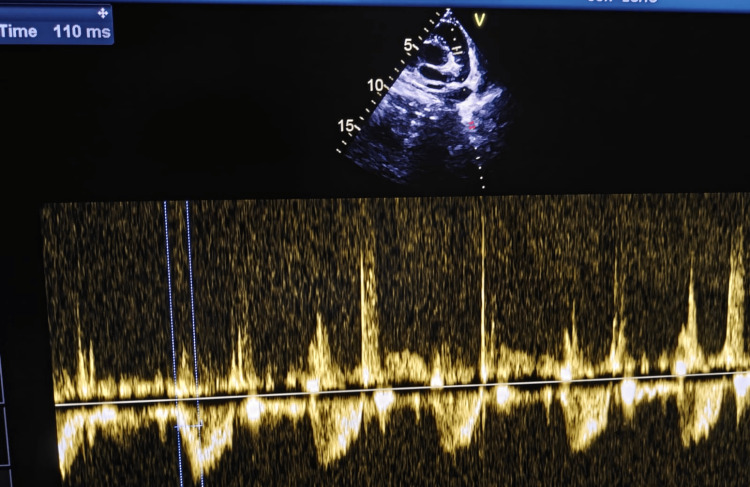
2D echo: high parasternal short axis (PSAX) view Mean pulmonary artery pressure (mPAP) = 90 − (0.62 × acceleration time) mPAP = 90 − (0.62 × 110) = 21.8 mmHg, i.e., normal (Normal mPAP in children >3 months is <20–25 mmHg).
